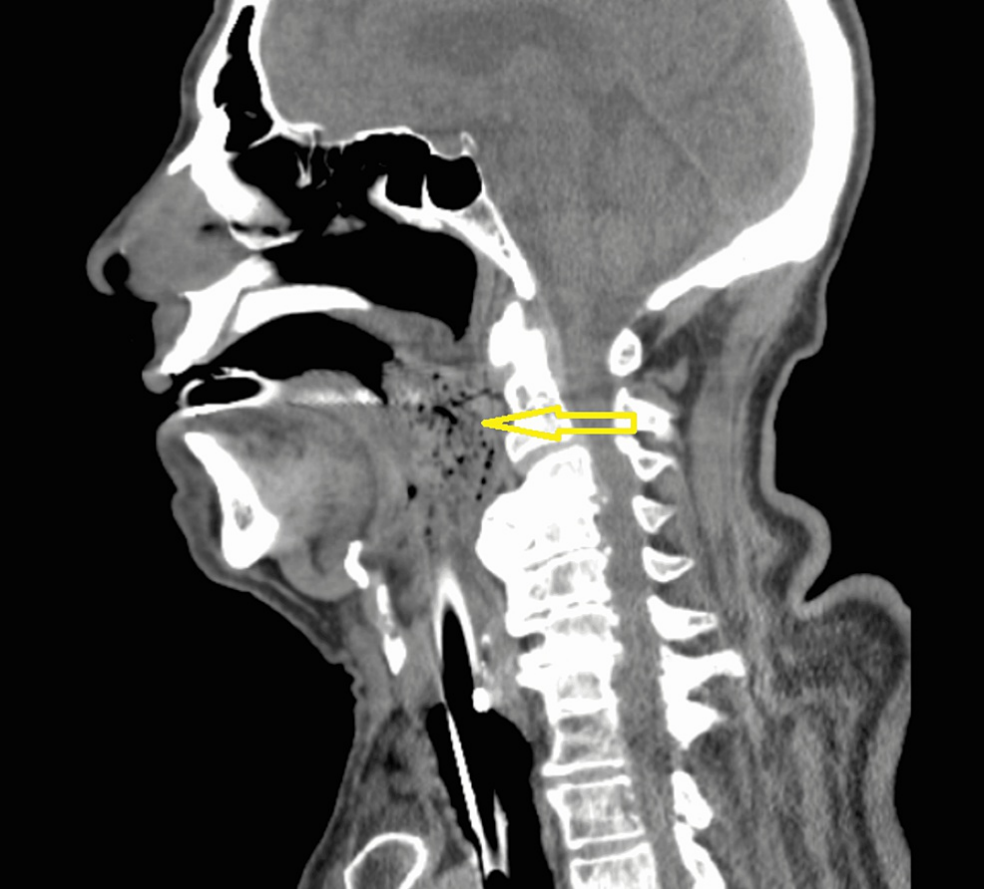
Computed tomography scan of the neck. Sagittal section showing 5.3 cm craniocaudal abnormal material.
